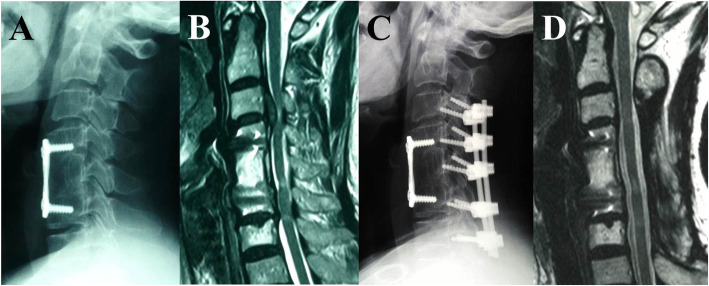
A 63-year-old woman developed two-level ASD with myelopathic symptoms at the 8th year after the primary surgery and underwent PDF with instrumented fusion as a revision surgery. a Lateral radiograph after the primary surgery showing that ACCF was performed at C5. b MRI before revision surgery showing a large disc extrusion behind the C3 vertebral body accompanied by ISI on the spinal cord at C3–C4 and C6–C7. c Lateral radiograph after revision surgery showing that posterior decompression with instrumented fusion was performed at C3–C7. d MRI at 2nd year postoperatively showing resorption of the herniated disc and release of spinal cord compression but with residual ISI at C6–C7.
